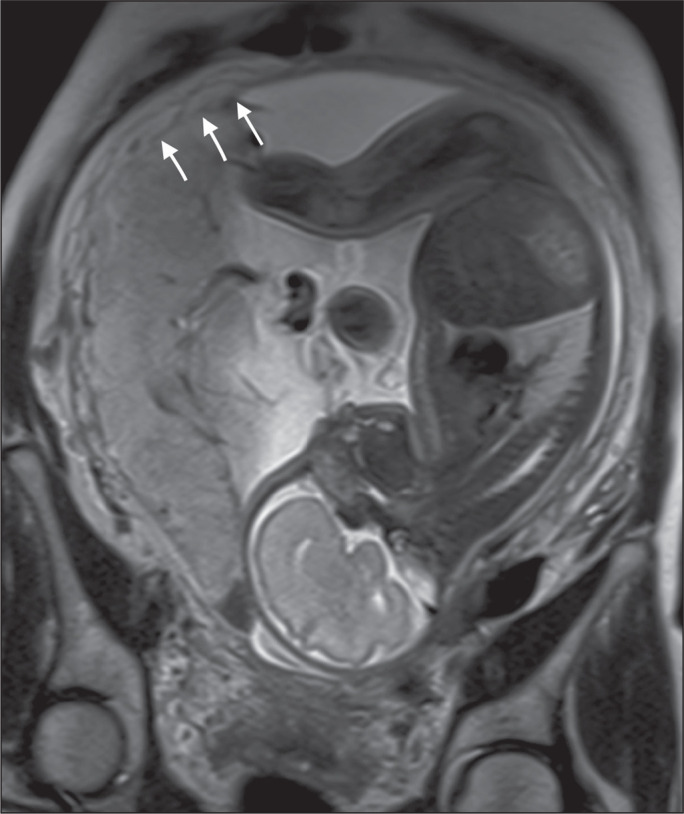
A 39-year-old patient. Coronal T2-weighted HASTE sequence showing
